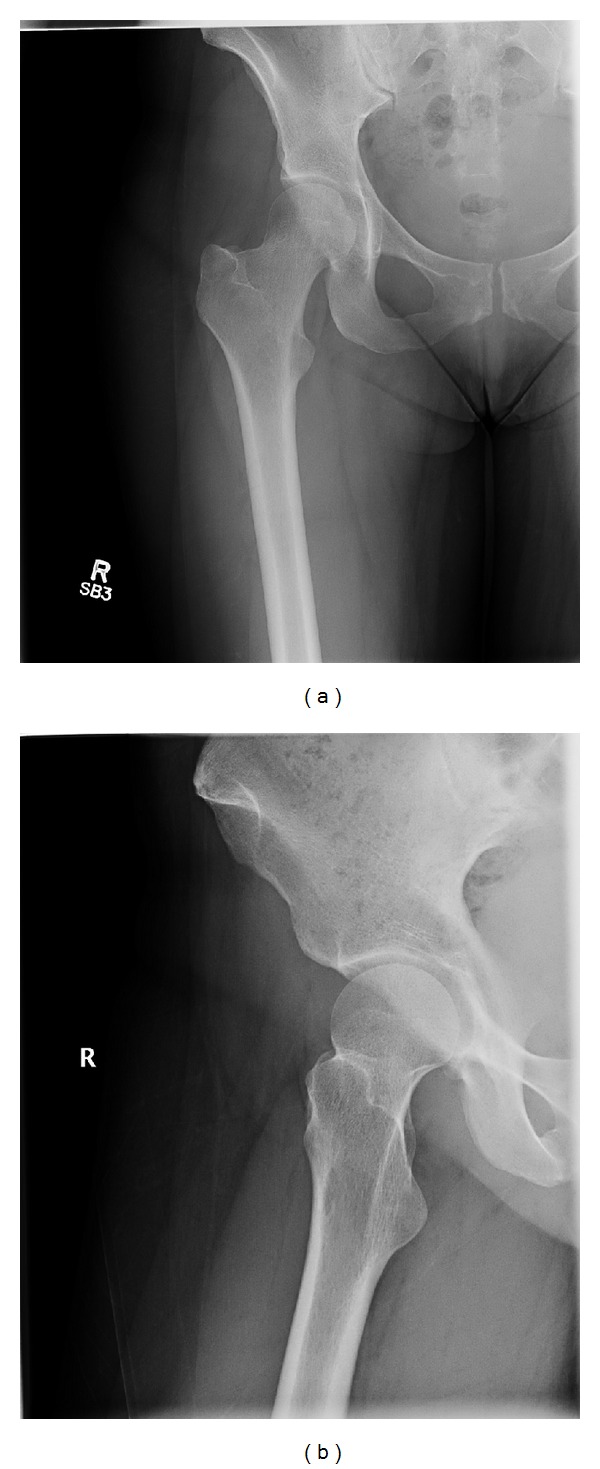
Frontal (a) and internal (b) rotation plain radiographs of the right hip reveal no discrete osseous lesion, cortical destruction, or soft-tissue mass.
